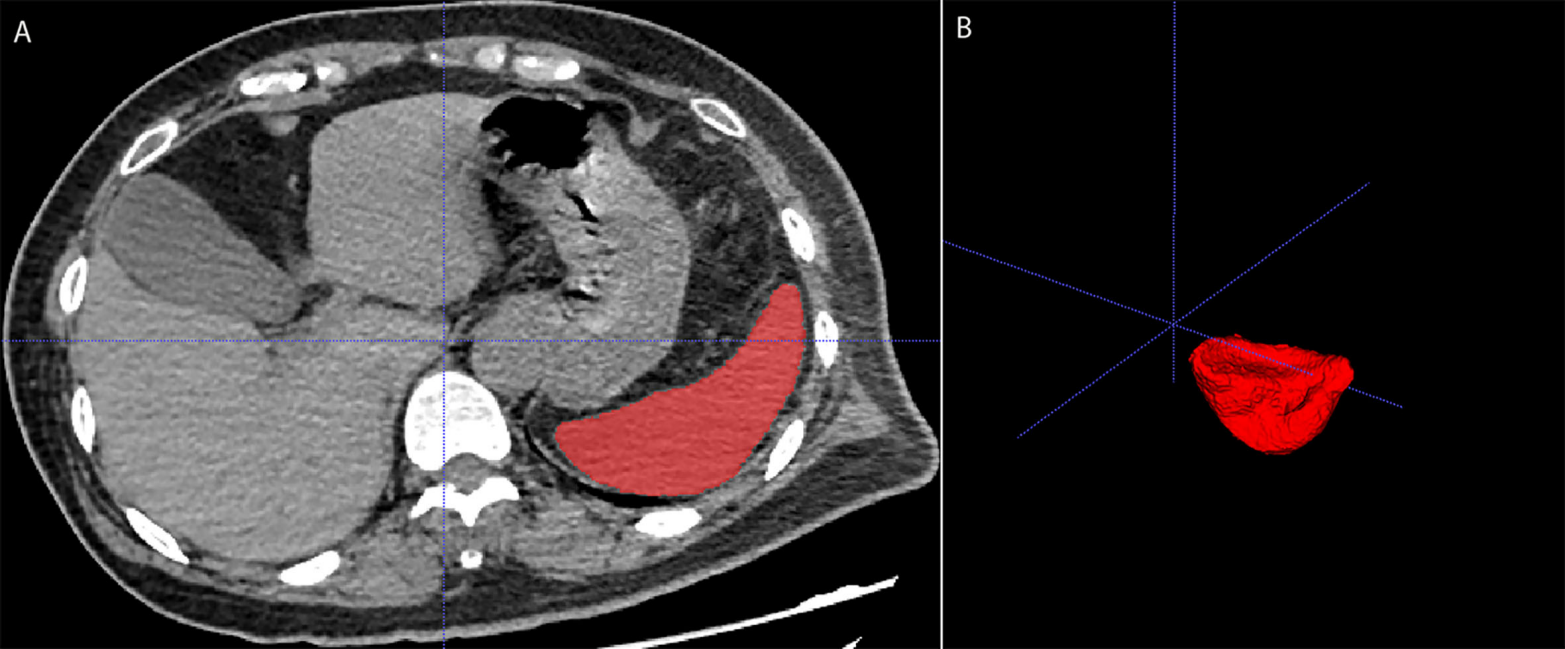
Measurement of splenic volume on unenhanced chest computed tomography using ITK-SNAP software. (A) showed the spleen segmentation, while (B) presented 3D model of the spleen.
